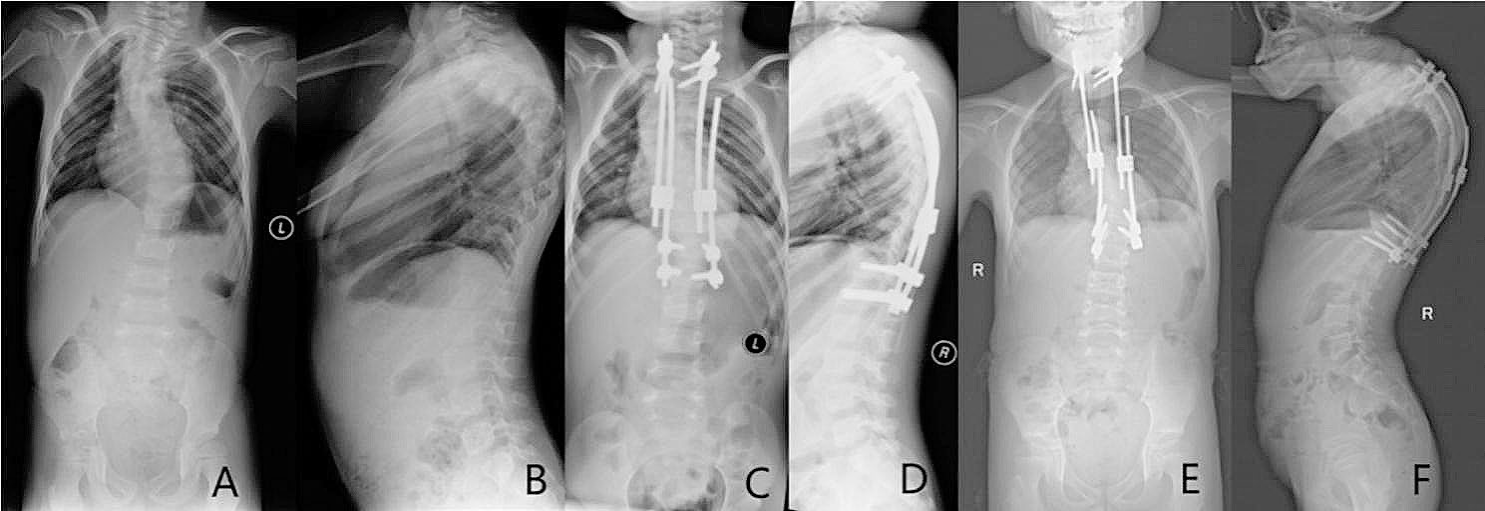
6-year-old girl, syndromic early onset scoliosis; A, B, Preoperative X-ray; C, D, Use traditional bilateral growing rod technique, after first operation, X-ray; E, F, Seventy-seven months (six lengthening) follow-up
